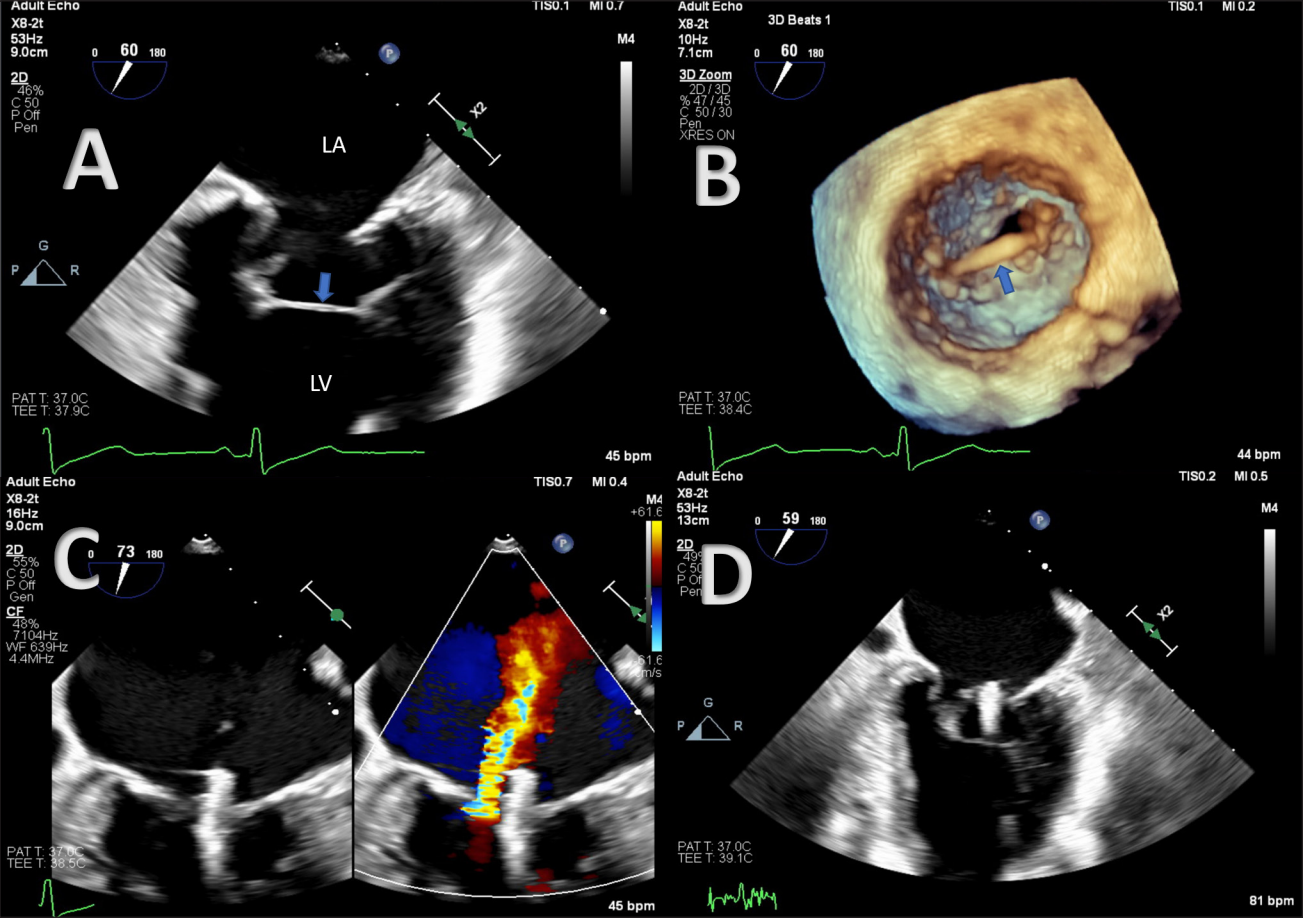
(A) Two-dimensional transesophageal echocardiography (TEE) (mid-esophageal 2-chamber view) shows a transverse chord-like structure (blue arrow) connecting the papillary muscles. (B) Three-dimensional TEE of the mitral valve highlights the transverse tendon (blue arrow). The procedure was successful with (C) residual mild mitral regurgitation, and (D) the tendon remained intact. LA: left atrium; LV: left ventricle.
